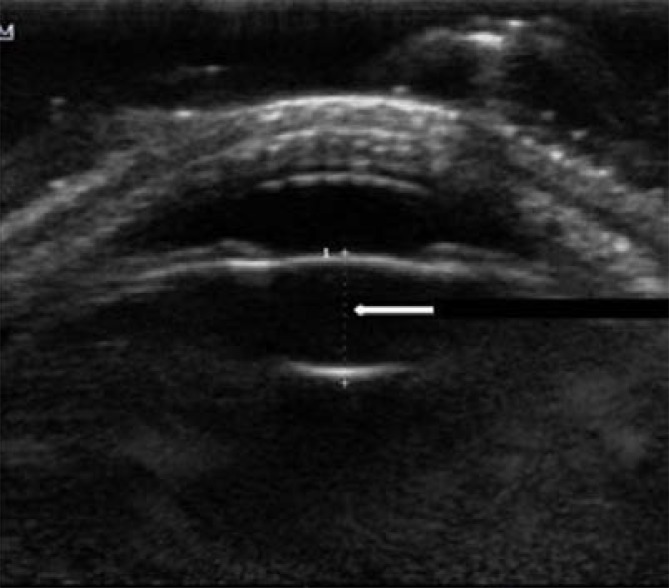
B-mode sonographic image of the eye (axial plane) showing measurement of the lens thickness (white arrow) as the anechoic space between the echogenic anterior and posterior lens capsules (delimited by cursors).
